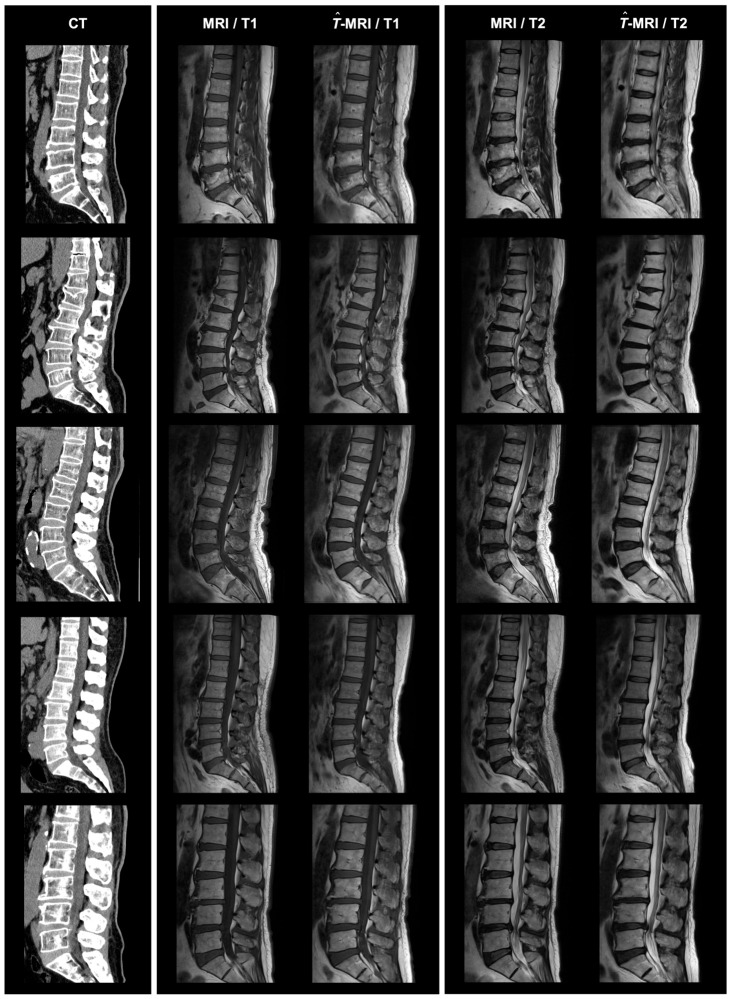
Representative sagittal slices comparing CT, MRI, and $\hat{T}$-MRI. Columns show CT, MRI/T1, $\hat{T}$-MRI/T1, MRI/T2, and $\hat{T}$-MRI/T2. Each row represents a different case. $\hat{T}$-MRI preserves CT-consistent anatomy while providing MRI-like soft-tissue contrast in qualitative visual assessment.
